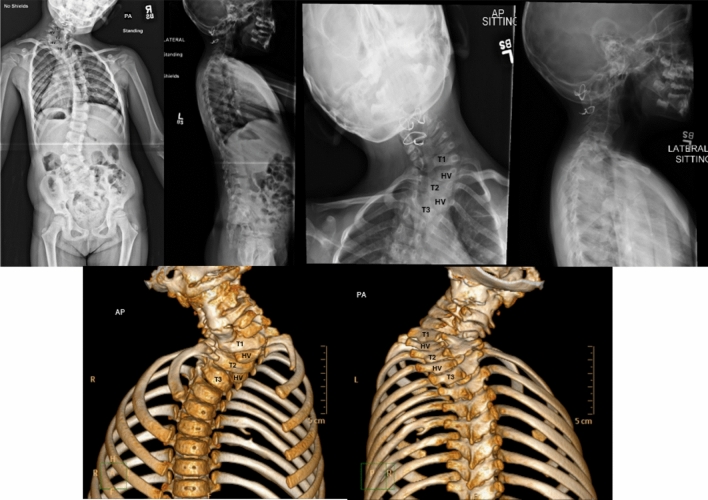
Case 3 Preoperative PA and lateral X-ray and reconstructed CT of a 4-year-old female with Klippel–Feil syndrome, C1–C2 instability, and congenital scoliosis. *HV* Hemivertebra
